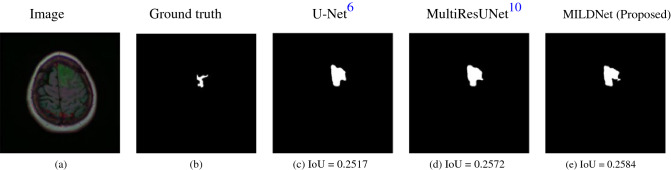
Segmenting a very challenging MRI^15^ image (a) having indistinguishable boundaries between the background and the foreground, with (b) being the ground-truth. All models including the proposed approach have over-segmented the image (c–e).
